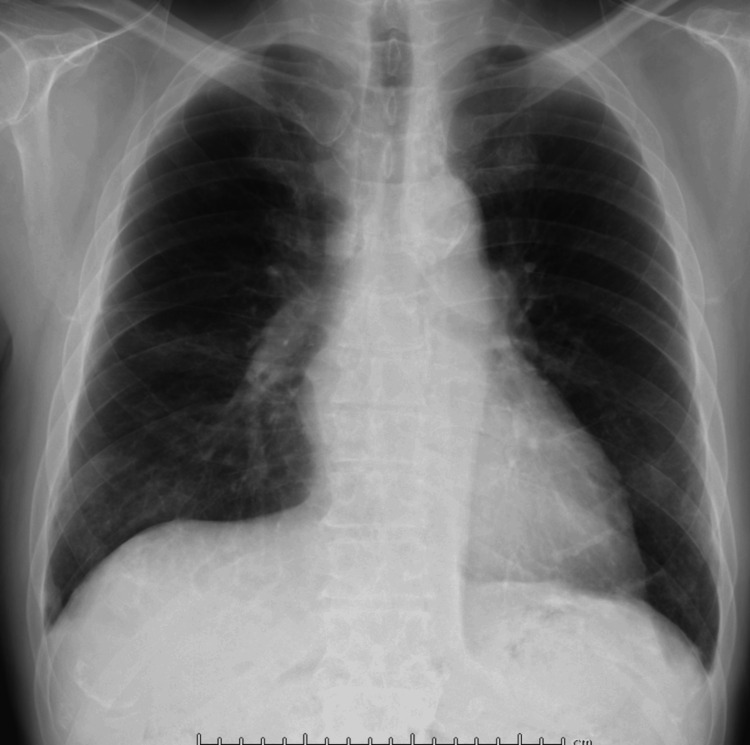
Chest radiograph A chest radiograph demonstrating ground-glass opacities in the right lower lung field. No evidence of cardiomegaly is observed.
